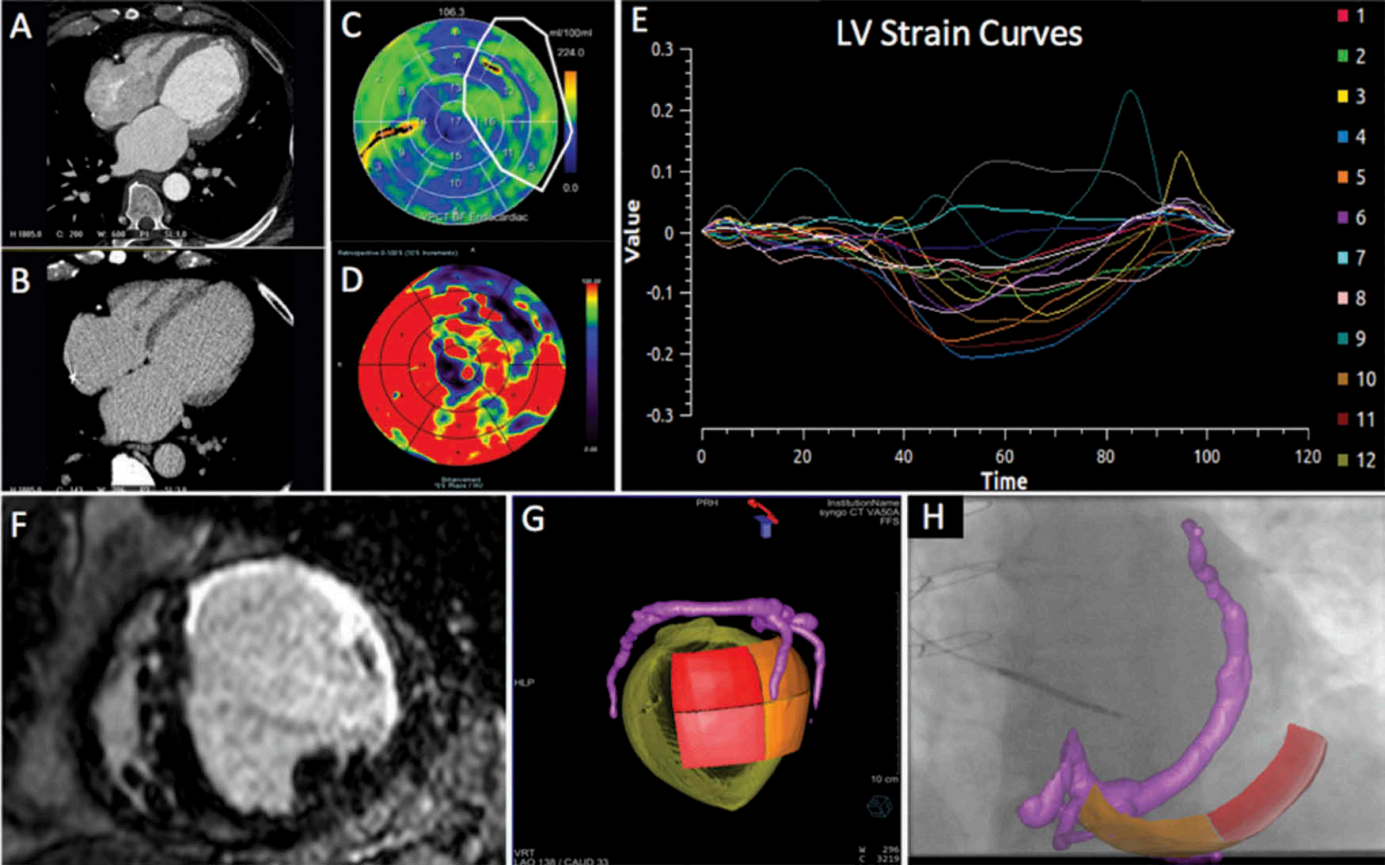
Series of (Dual Energy CT) DECCT-derived scar and image overlay of the coronary sinus and optimal target segment derived from CT strain measurements from one patient: Retrospective CCT demonstrating calcification in a left anterior descending (LAD) and circumflex territory infarct (A). Dual-energy CCT demonstrating subtle ventricular scar in the LAD and circumflex territory (B). Late iodinated enhancement plotted on American Heart Association (AHA) 17-segment bull’s-eye plot suggesting scar in the LAD and circumflex territory but also artifact from an existing RV pacing lead in the basal to mid-antero-septum (C). First pass iodine uptake plotted on an AHA 17-segment bull’s-eye plot showing what we believe to be residual iodine predominately in the LAD and circumflex territory (D). CCT-derived dyssynchrony curves calculated by myocardial strain (E). Cardiac magnetic resonance short axis image of the mid-LV showing late gadolinium enhancement of the same patient taken 2 years prior to any device implantation for comparison purposes (F). Pre-procedure DECCT-derived coronary sinus segmentation fused with latest mechanical activating segments determined from DECCT-derived strain (G) co-registered and overlaid onto live fluoroscopy using fusion software (H). Reproduced with permission from Oxford University Press.
